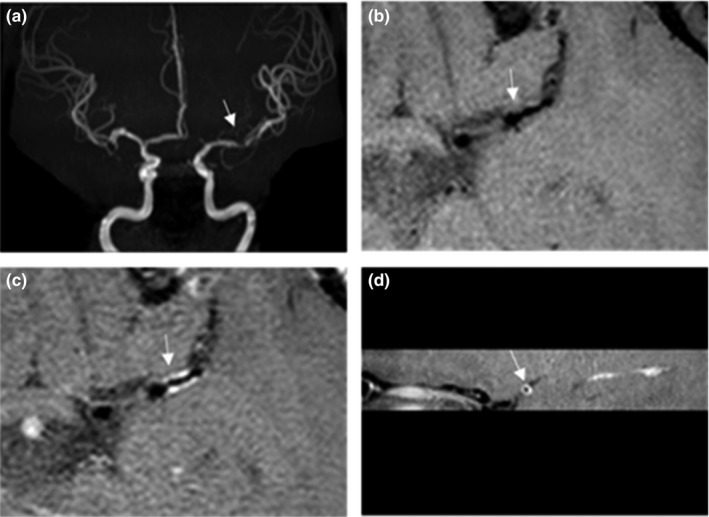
(a) Female, 35 years old, 3D‐TOF MRA shows focal lesions in the M1 segment of the left middle cerebral artery, (b and c) HR‐MRI showed that the wall of the tube was uniformly thickened and strengthened, and (d) the lumen is moderate–severe stenosis
